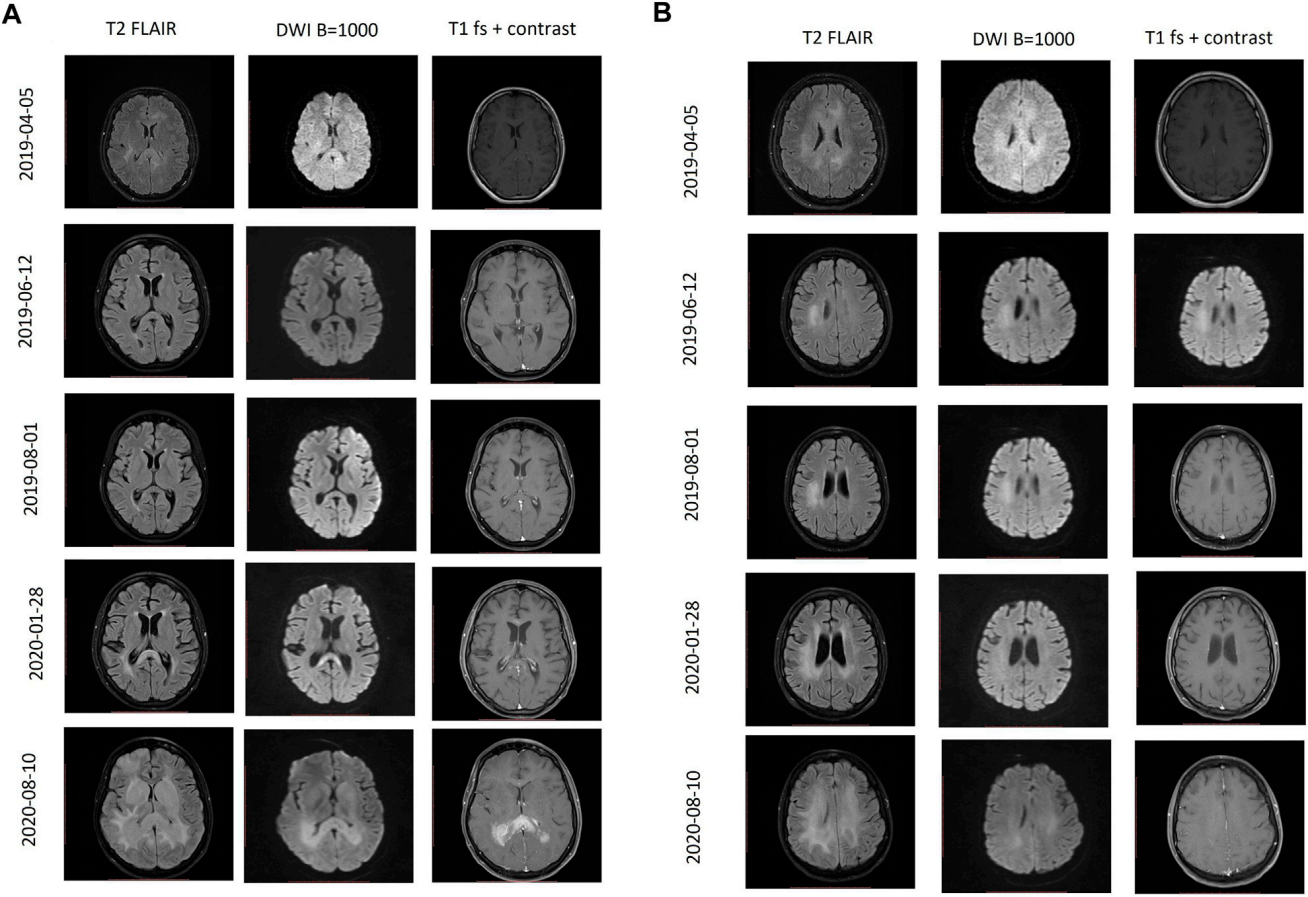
Follow-up MRI scans during different periods of treatment. (A) T2 FLAIR, DWI, contrast-enhanced T1 axial MR images at the level of basal ganglia. (B) T2 FLAIR, DWI, contrast-enhanced T1 axial MR images at the level of frontal horns of the lateral ventricles. 05 April 2019 Primary MRI scan. 12 June 2019 MRI follow-up examination after completion of first-line chemotherapy shows revisal of the hyperintensity lesions in the right parietal lobe, corpus callosum, and corona radiata. 01 August 2019 MRI follow-up examination demonstrates the progression of the lesion in the right parietal lobe. No additional lesions were found. 28 January 2020 MRI follow-up examination demonstrates enlarged enhancing lesion of the dorsal part of the corpus callosum with restricted diffusion. 10 August 2020 MRI follow-up examination demonstrates T2 hyperintense contrast-enhancing masses of the dorsal part of corpus callosum extending to basal ganglia with significantly restricted diffusion, perifocal edema, and compression of the ventricle system.
